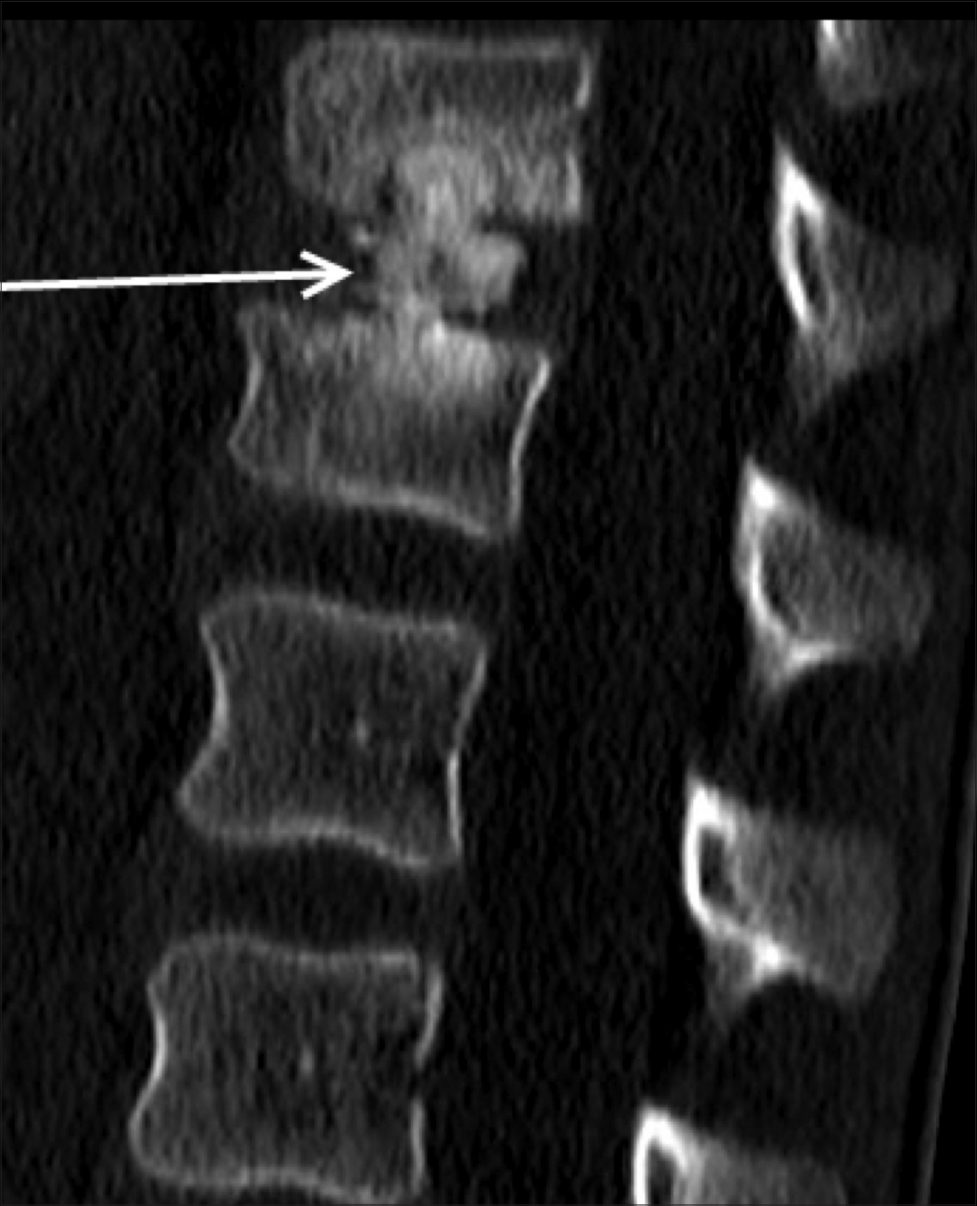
Reformatted sagittal CT scan shows swelling and calcification of the D12/L1 nucleus pulposus with intravertebral herniation (arrow), without endplate destruction
